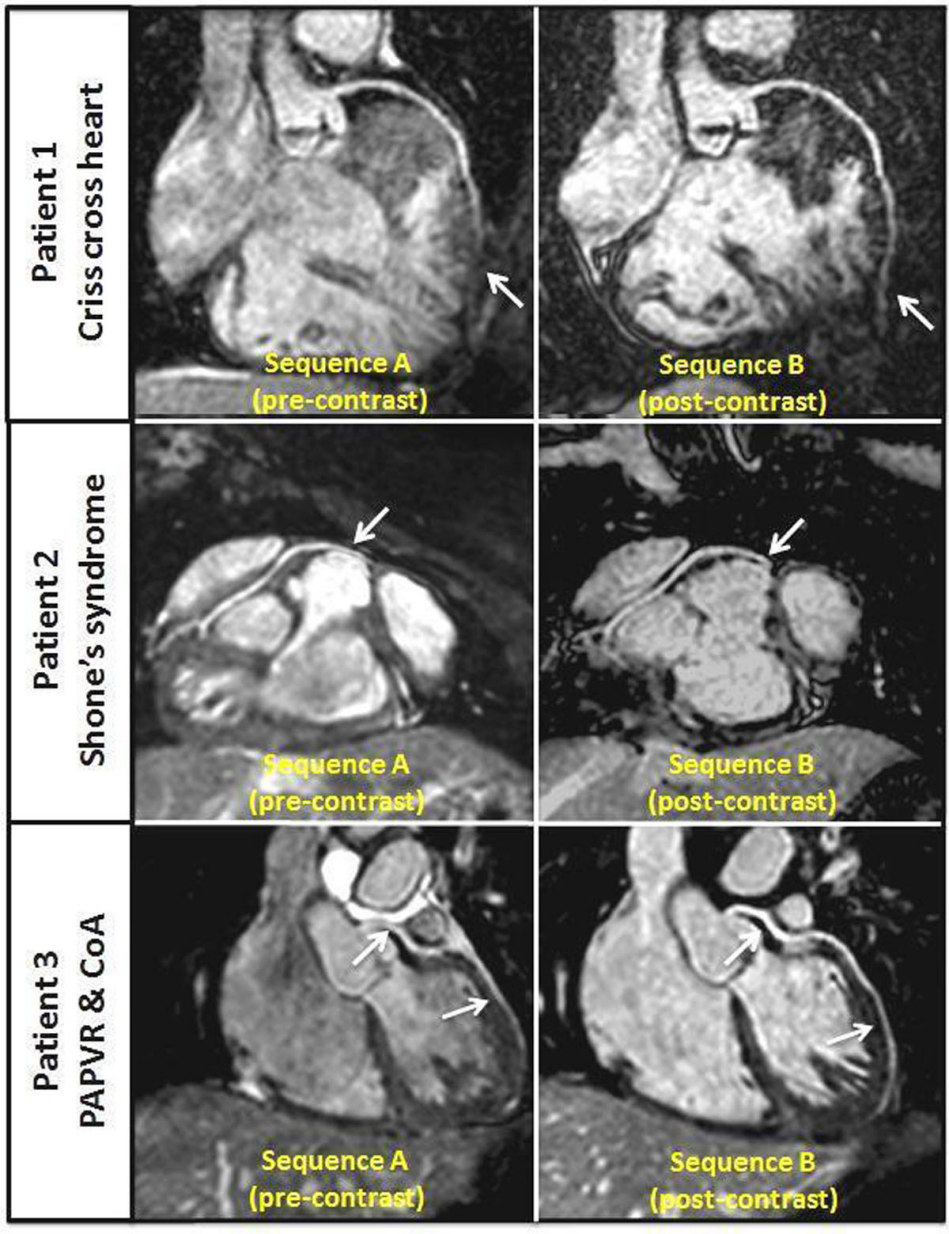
Whole-heart 3D steady-state free precession coronary magnetic resonance images from three patients reformatted using Soap-Bubble software. Left-hand panels - sequence A (pre-contrast). Right-hand panels - sequence B (post-contrast). Arrows point to coronary segments with improved visualisation after contrast. PAPVR, Partial anomalous pulmonary venous return. CoA, aortic coarctation.
